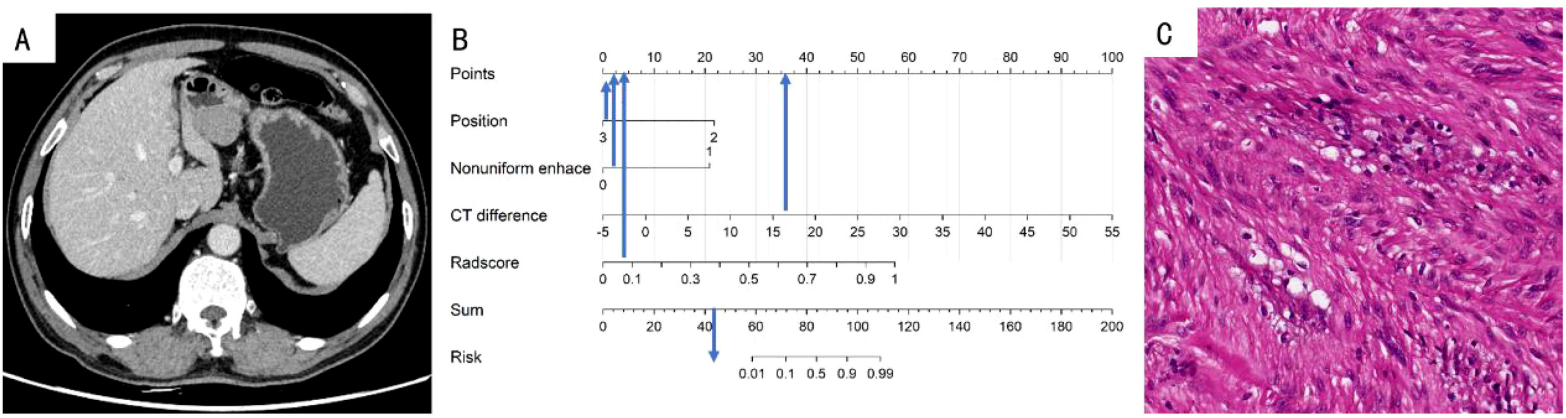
(A) A 70-year-old woman with GS. The axial CT image demonstrated a 3.4-cm-sized tumor in the antrum of the stomach. (B) Total points of the tumor was 44, risk value was far less than 0.1 and it was classified as GS by the nomogram. (C) Pathological HE (×200) of the tumor.
